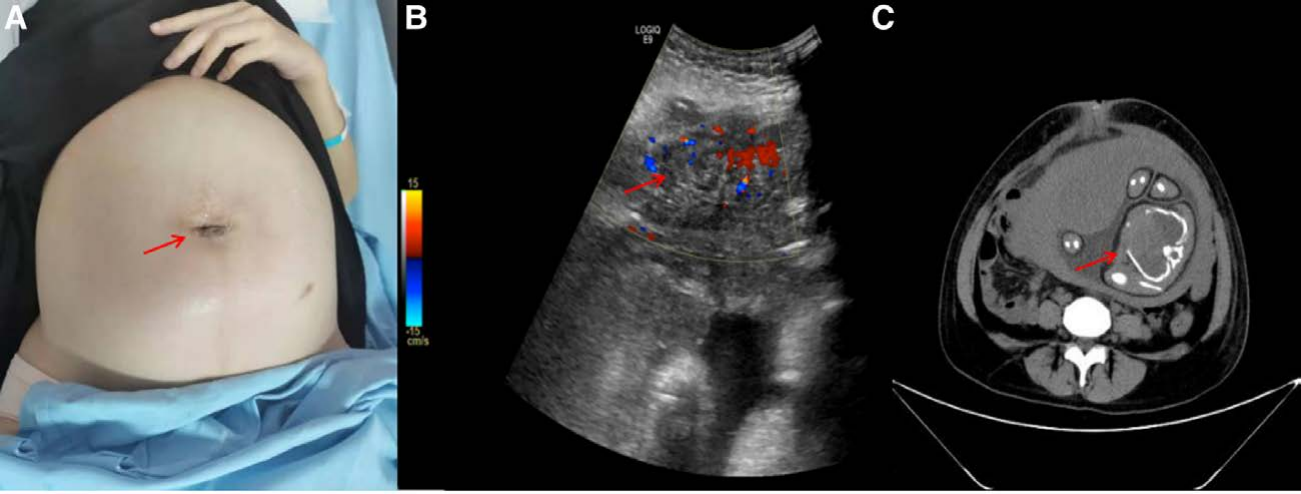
Body surface image (A) showing redness and swelling around the umbilicus with a size of about 5 cm. Color Doppler Ultrasound (B) showing the abdominal wall around the umbilicus was descending and mixed masses, with an area of about 18.4 × 43.1 cm, extending down to the suprapubic bone. Abdominal computed tomography (CT) (C) showing the uterus is enlarged, a fetus is seen in the uterine cavity, and there is no umbilical cord echo around the fetal neck; fetal position: head position, the fetal head is above the symphysis pubis. Stable fetal vital signs.
